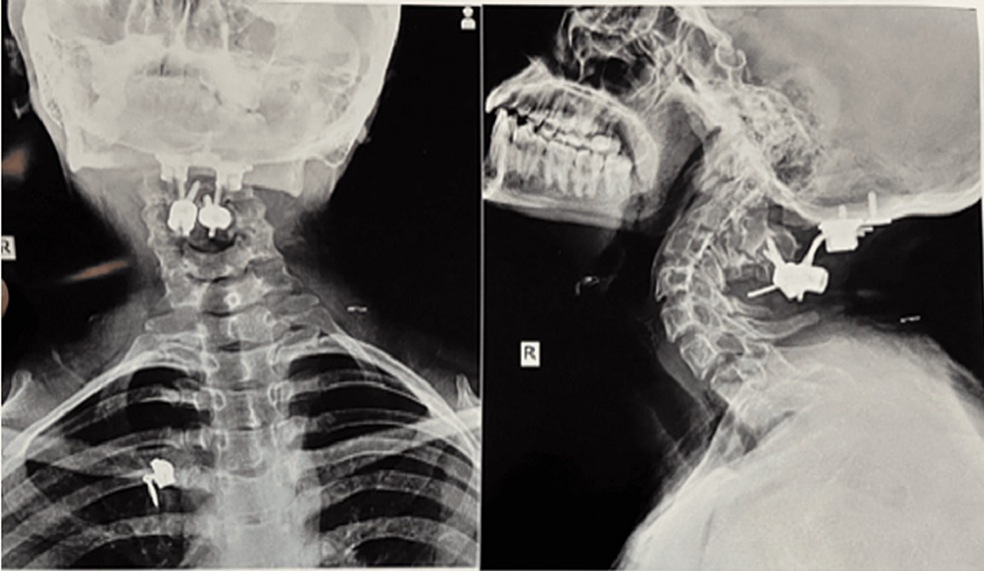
X-ray view depicting skeletal abnormality.
